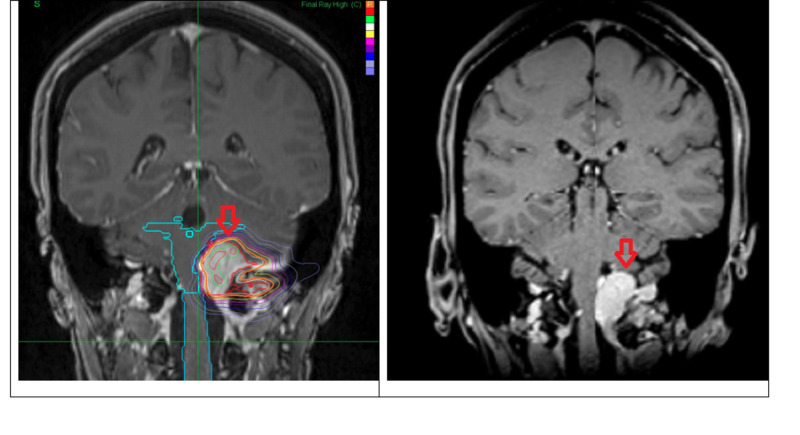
Left: pre-treatment coronal view of T1 contrast-enhanced MRI. Right: eight years post-treatment showing a 30% decrease in tumor volume and involution of mass effect on the brainstem.
